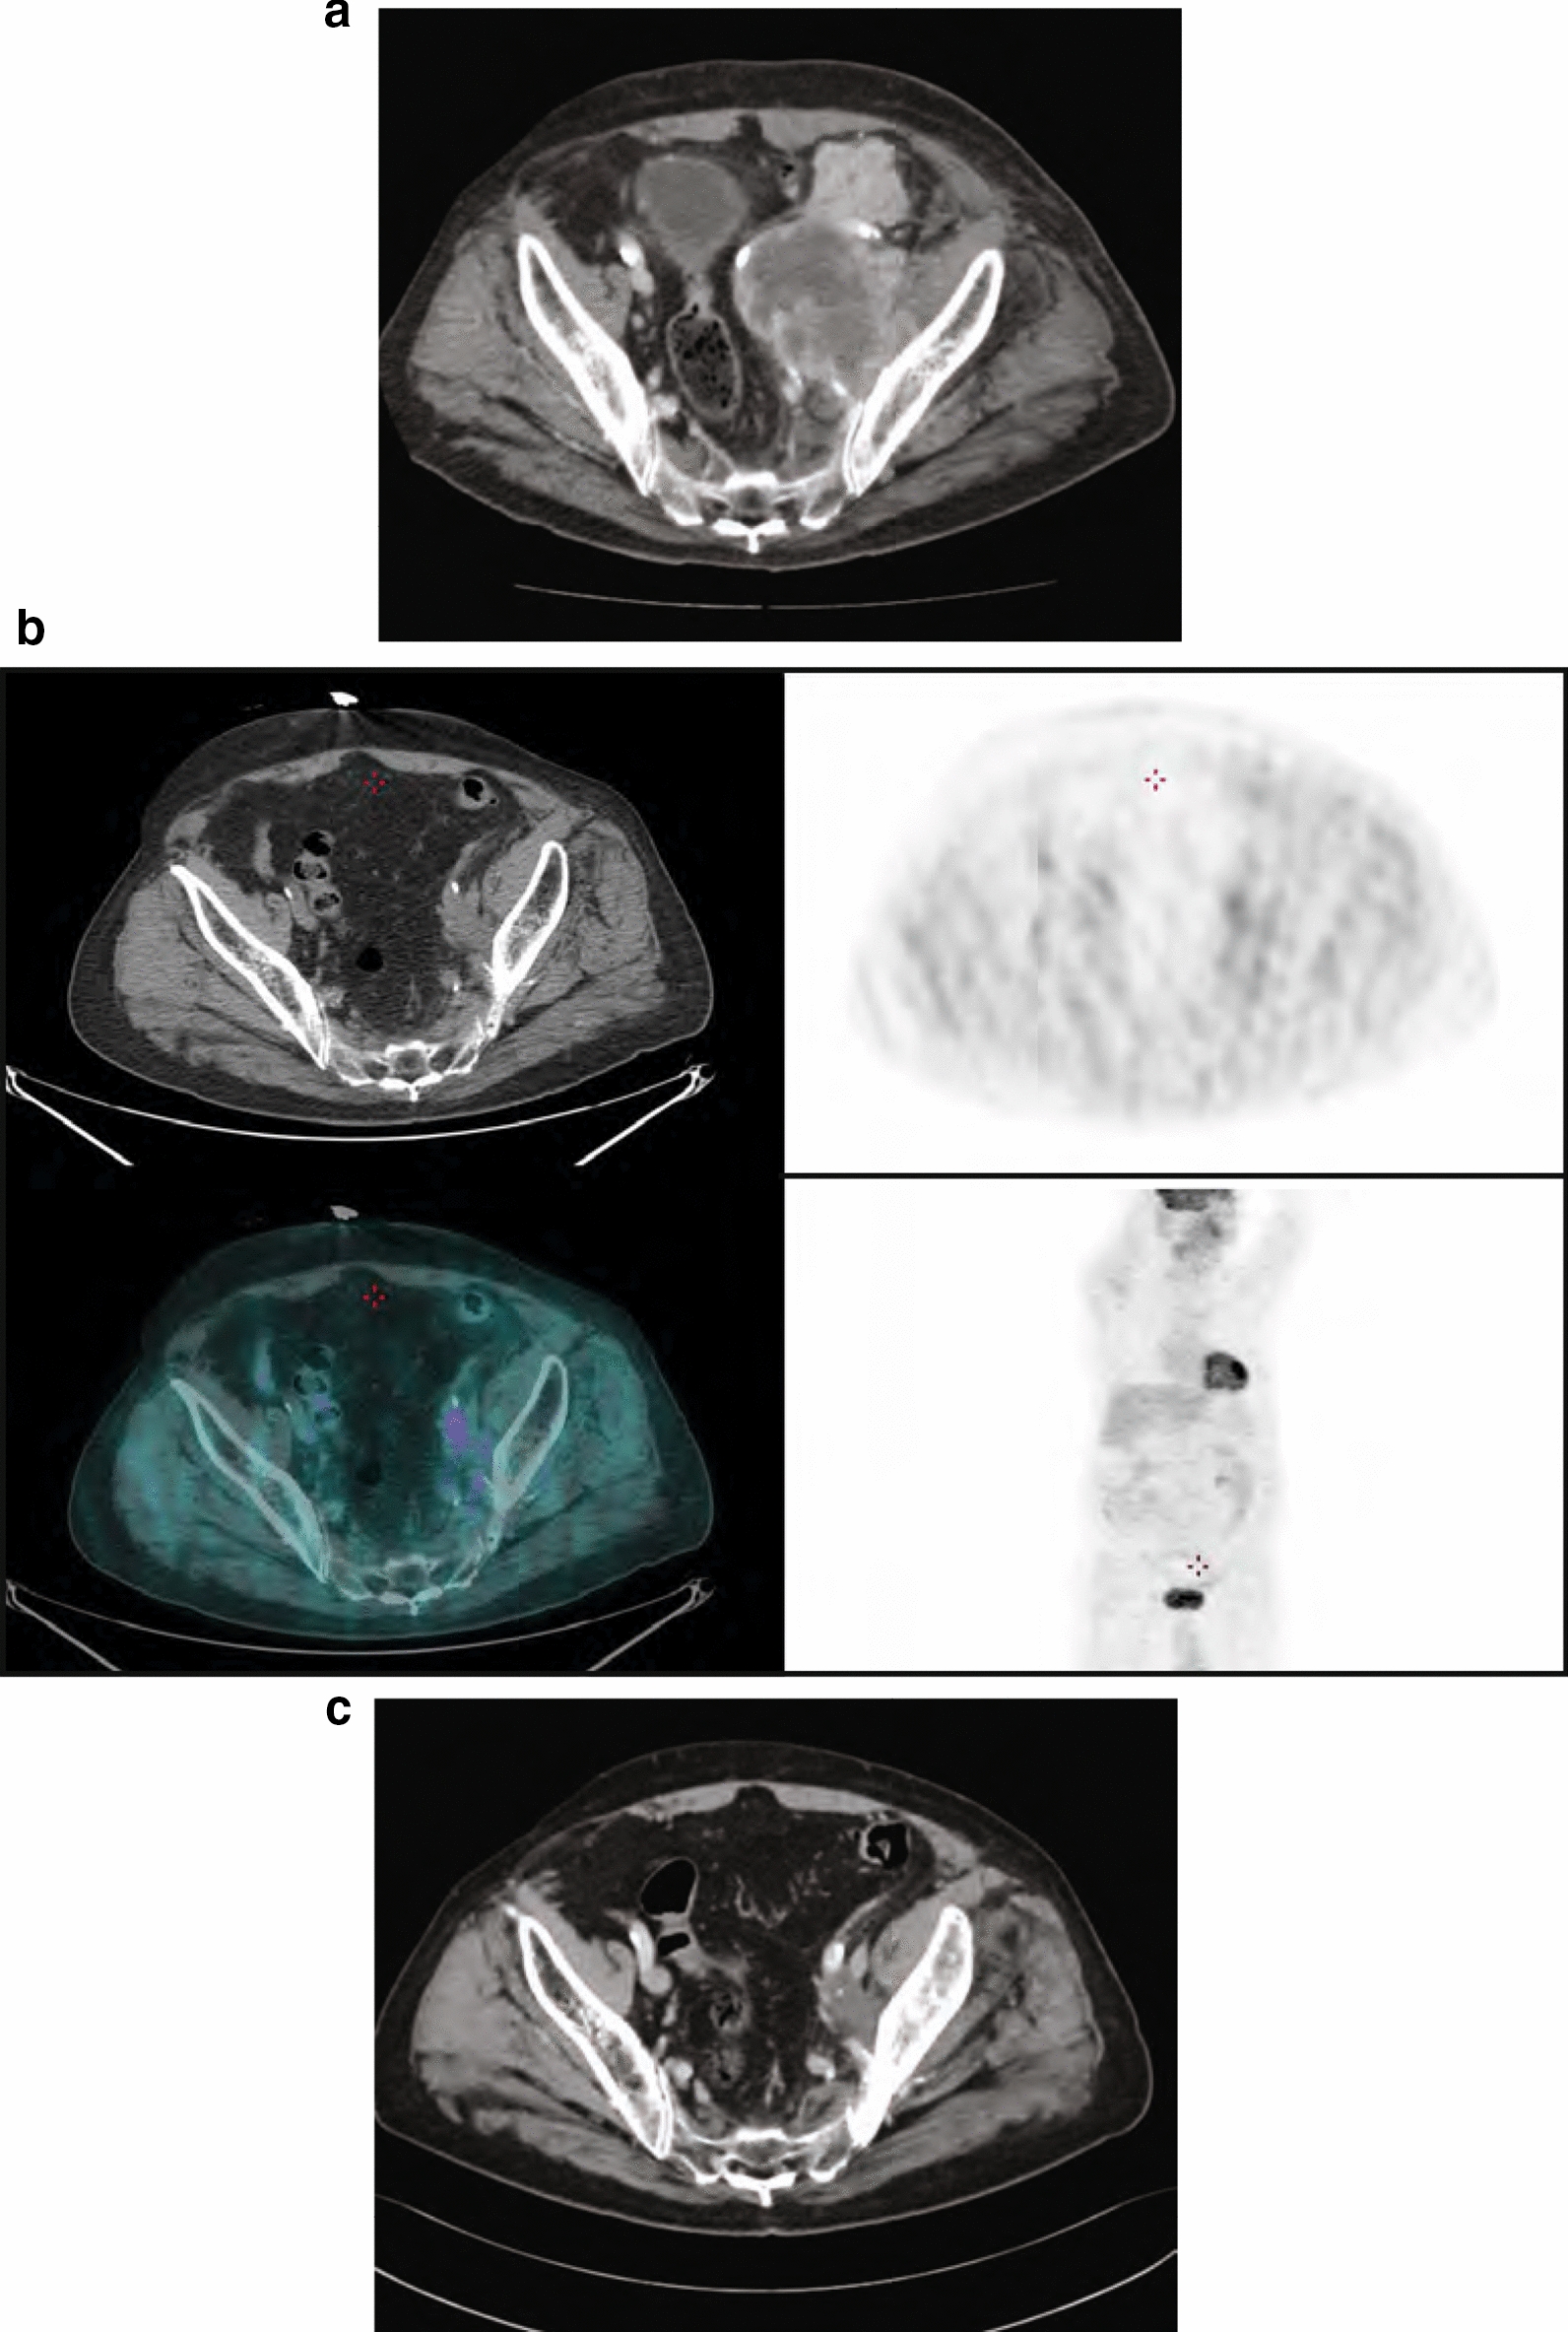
CT/PET-CT scans from a patient with metastatic Merkel cell carcinoma treated with avelumab. a Progressive disease at baseline (January 2017), b CR at 20 months after starting avelumab treatment (September 2018), and c CR in June 2020. Images were provided by Dr. Ciliberto. *CR* complete response, *PET-CT* positron emission tomography–computed tomography
